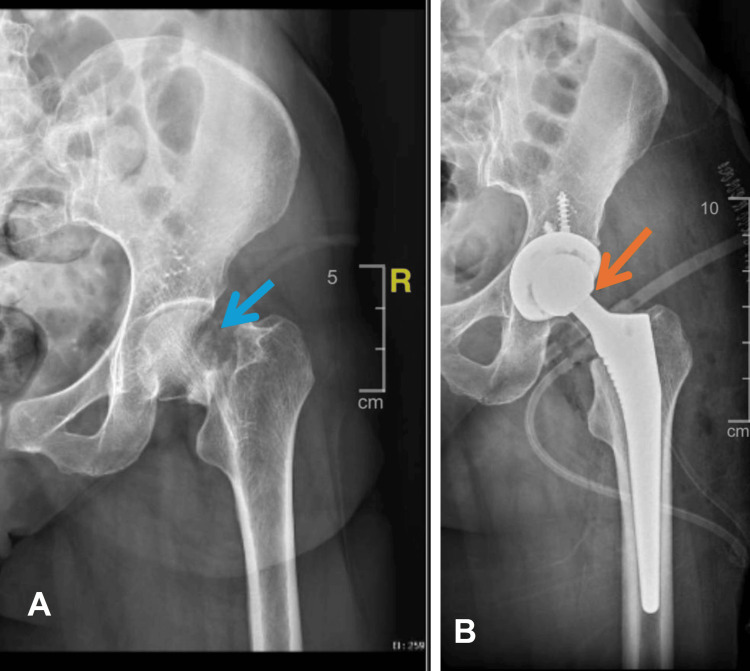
Preoperative radiograph (A) showing fracture of neck of femur (blue arrow) and postoperative (B) radiograph showing well-functional Latitud™ hip replacement system (orange arrow) implanted in a patient undergoing total hip arthroplasty
